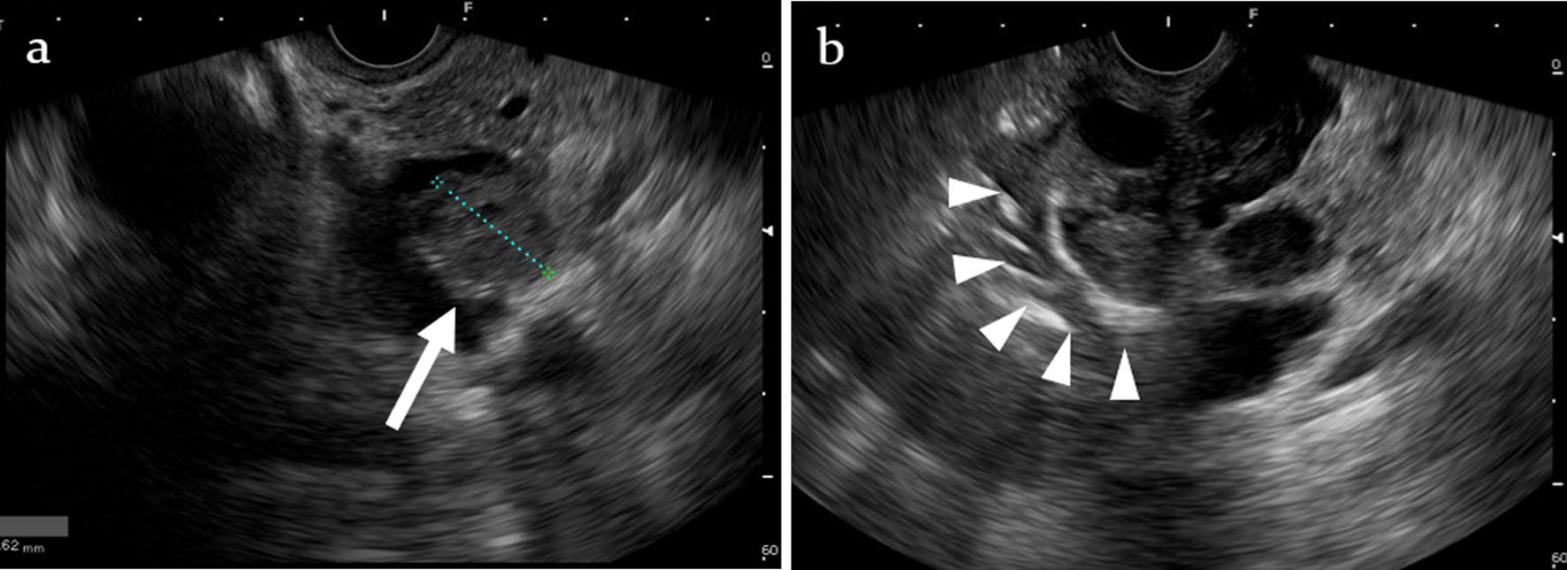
a Endoscopic ultrasound (EUS) revealed a multilobular cystic lesion with a 17 mm mural nodule (white arrow) in the pancreatic head. The blood flow signal in the mural nodule was not observed. b Prominent calcification with acoustic shadow was observed on part of the cyst wall (white arrowheads)
